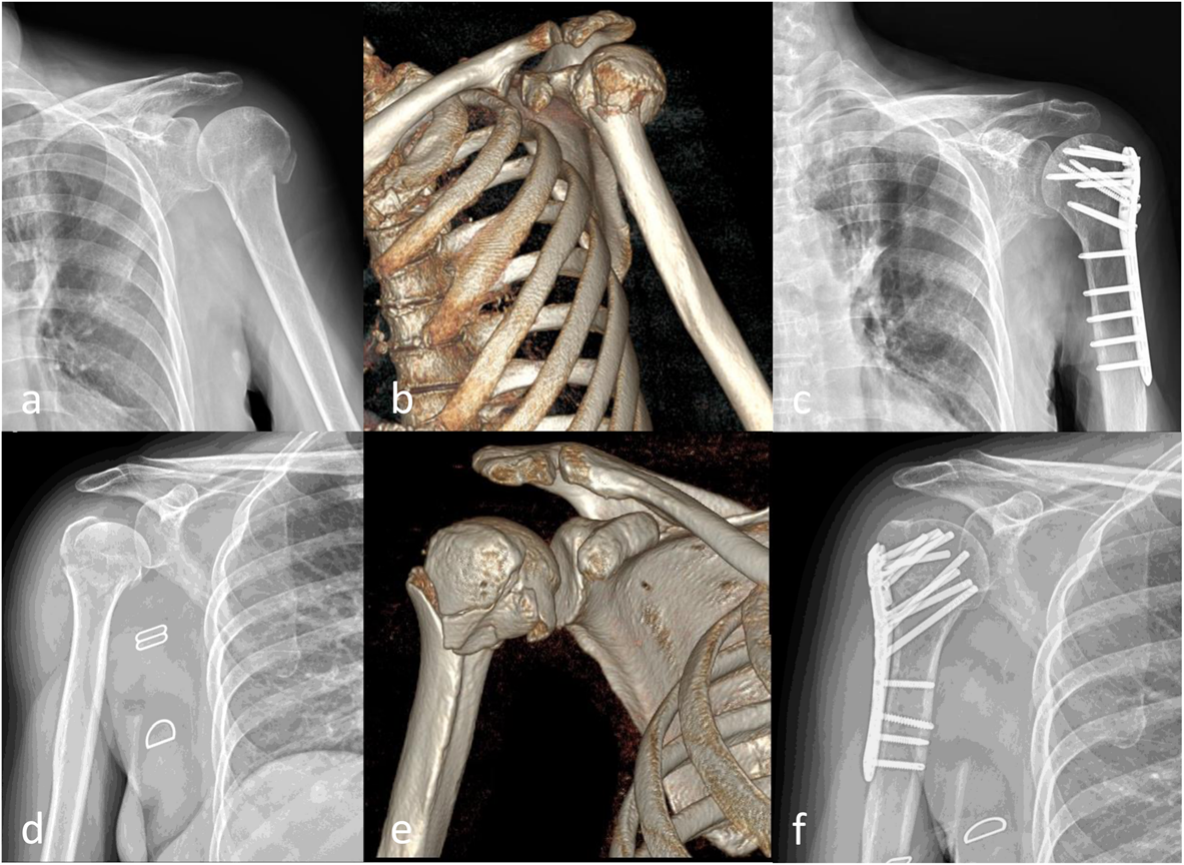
a Radiograph of a 79-year-old woman with Neer classification of three-part proximal humeral fracture. b Three-dimensional (3D) computed tomography (CT) reconstruction. c Radiograph at final follow-up 360 days after surgery with PHILOS plating. d Radiograph of a 72-year-old woman with Neer classification of three-part proximal humeral fracture. e CT scan with 3D reconstruction. f Radiograph at final follow-up 375 days after surgery with ALPS plating
